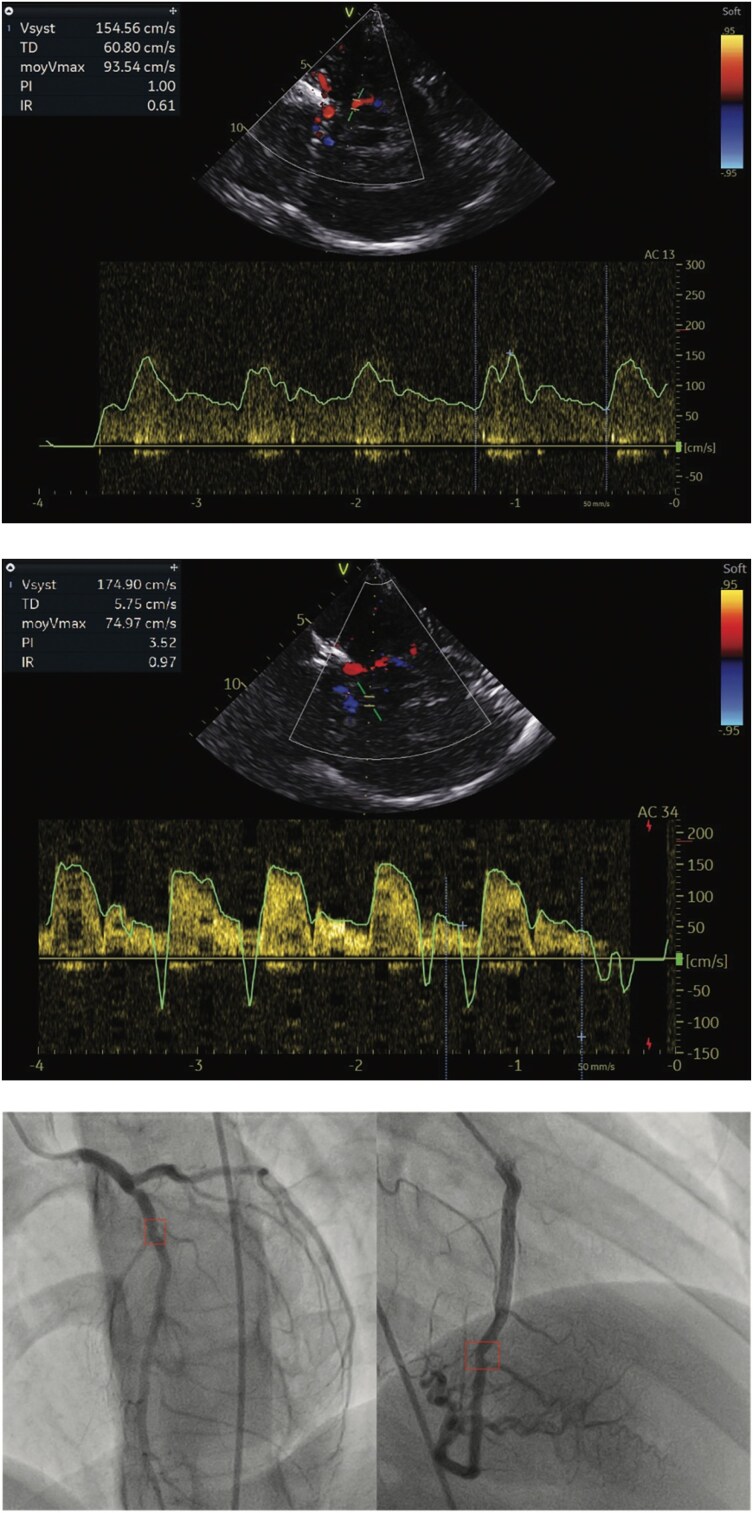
Transcranial Doppler revealed increased flow velocities in the middle cerebral artery. Image of coronary lesions.
